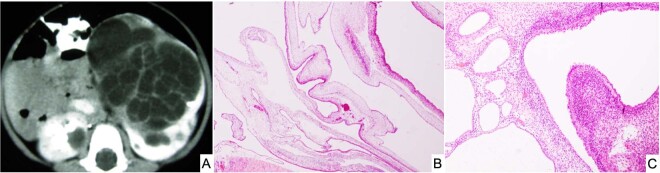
Cystic nephroma, pediatric type. A Circumscribed multicystic renal lesion in this CT image from a 2-year-old male who presented with an abdominal mass. A similar-appearing lesion on CT was present in the lung (PPB type I not shown). B Several delicate septal structures display architectural similarities to PPB type IR. C Multiple cysts in a fibrous stroma and one larger cyst with a hypercellular subepithelial mantle of spindle cells with a resemblance to PPB type I. Overtly sarcomatous elements were not present.
